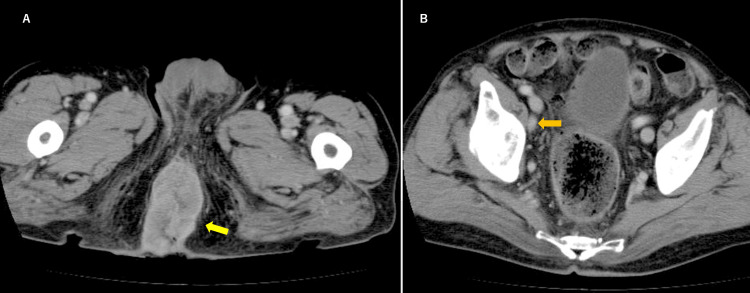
Contrast-enhanced CT images of Case 1 Contrast-enhanced CT showing anal canal cancer with suspected invasion into the perineum (A, yellow arrow) and metastasis to the right obturator lymph node (B, orange arrow).
